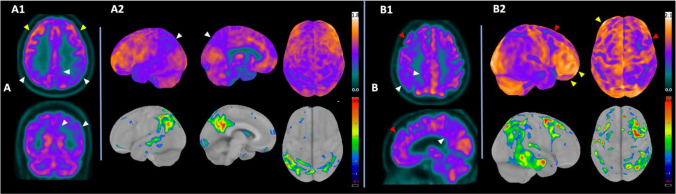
Typical pattern of neurodegeneration in Alzheimer’s Disease as seen on FDG-PET at different stages of progression in two individuals with predominantly amnestic presentation and confirmed cortical deposition of beta-amyloid plaques by [^11^C]-PiB PET. (A) Hypometabolism in the posterior temporal neocortex, temporoparietal associative cortex, posterior cingulate, and precuneus, slightly more evident on the left side, highlighted by white arrows. (A1) Images in axial and coronal planes, showing preserved frontal metabolism (yellow arrows). (A2) 3D-SSP* quantification images demonstrating in the top row the projection of metabolism on the cortical surface in left lateral, medial, and superior projections; in the bottom row, the projection of the Z-score of hypometabolic areas in relation to a normal control database by the same method is shown. (B) Patient in the moderate phase of the neurodegeneration process, with prefrontal hypometabolism (red arrows) in addition to hypometabolism in posterior temporoparietal regions (white arrows). Notable is the preservation of metabolism in polar and supraorbital frontal regions (yellow arrows), an important differentiation from other pathological processes (e.g., frontotemporal dementia). (B2) 3D-SSP* quantification images demonstrating in the top row the projection of metabolism on the cortical surface in right lateral and superior projections; in the bottom row, the projection of the Z-score of hypometabolic areas in relation to a normal control database is shown.
